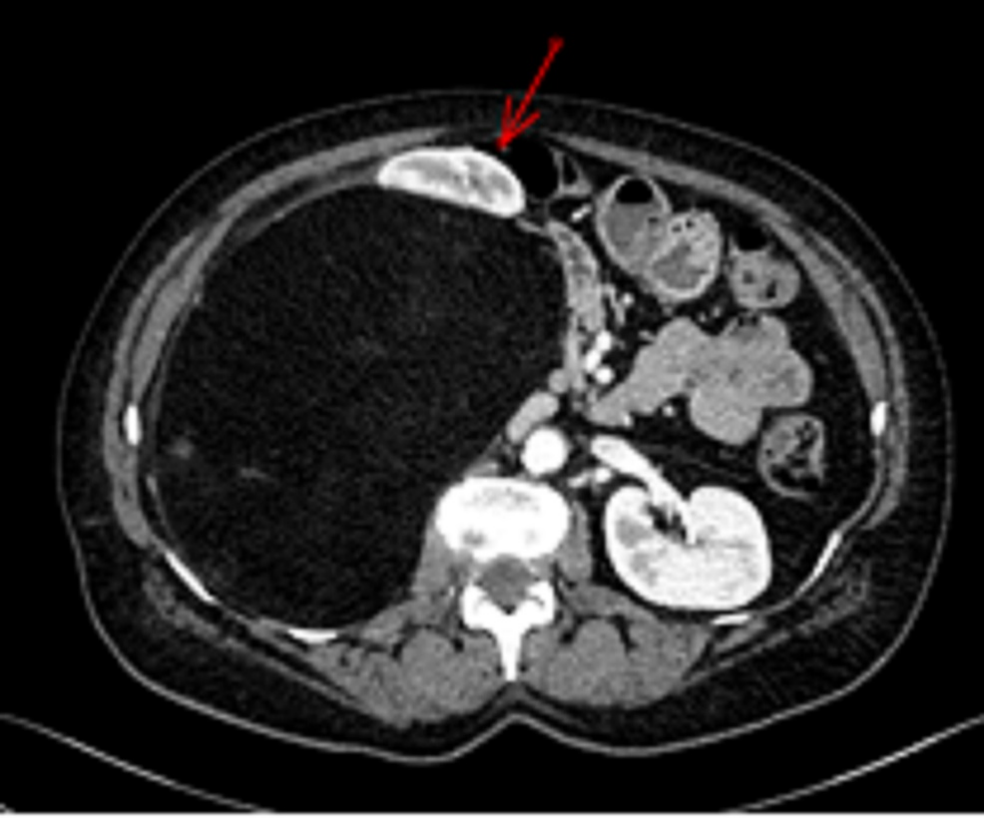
Case 1. Contrast-enhanced abdominal CT scan This shows a retroperitoneal adipocytic mass displacing the right colon and kidney to the left (red arrow).
